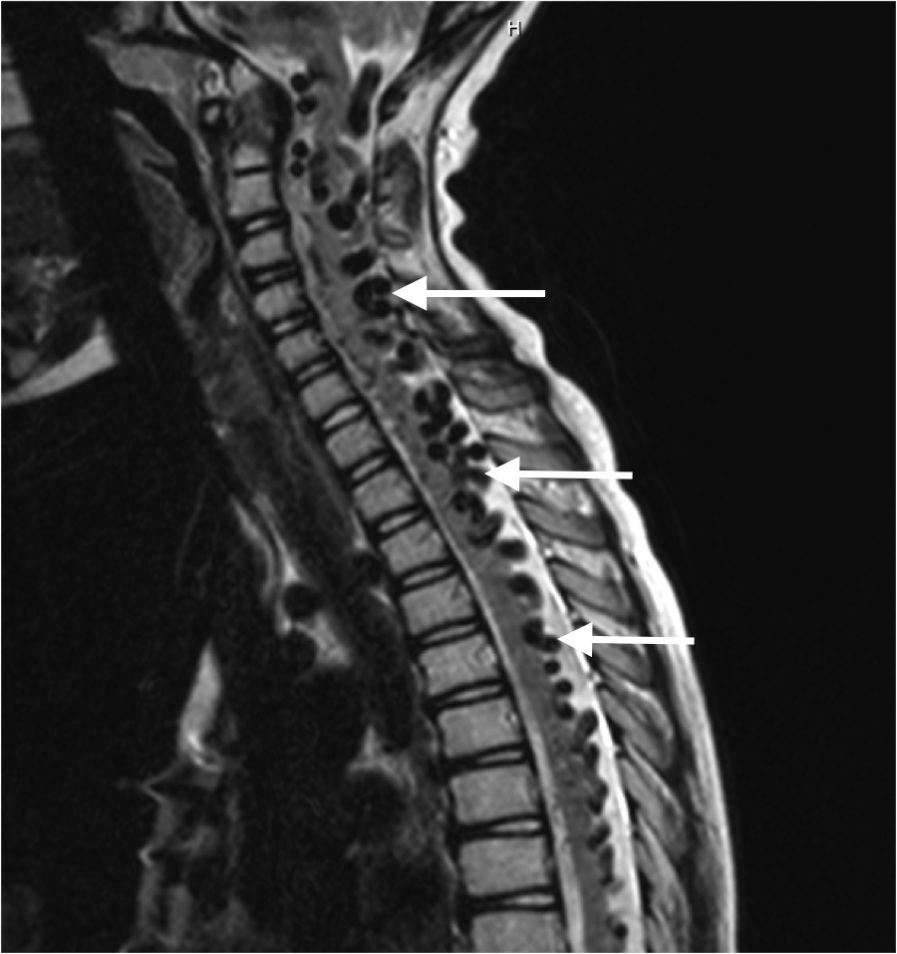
Sagittal magnetic resonance imaging showing serpiginous veins (white arrows) throughout the cervicothoracic spine
